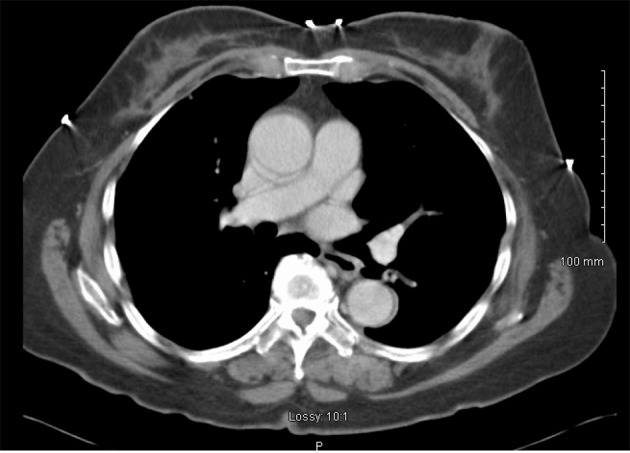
CT scan image after treatment demonstrating complete resolution of pleural effusion and nodularity.
